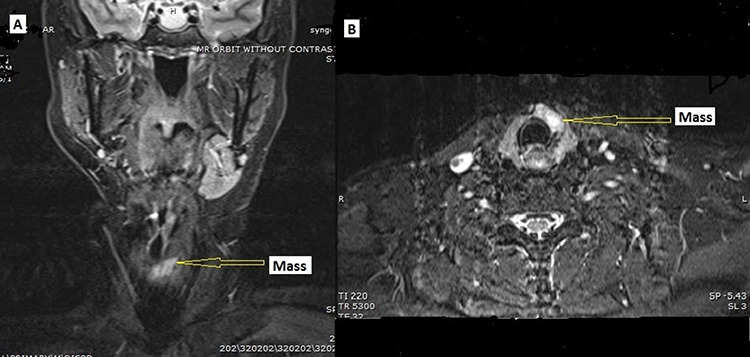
MRI. (A) Coronal and (B) axial, STIR image demonstrating hyperintense mass lesion (arrow pointing) within the isthmus extending into the left thyroid lobe.
